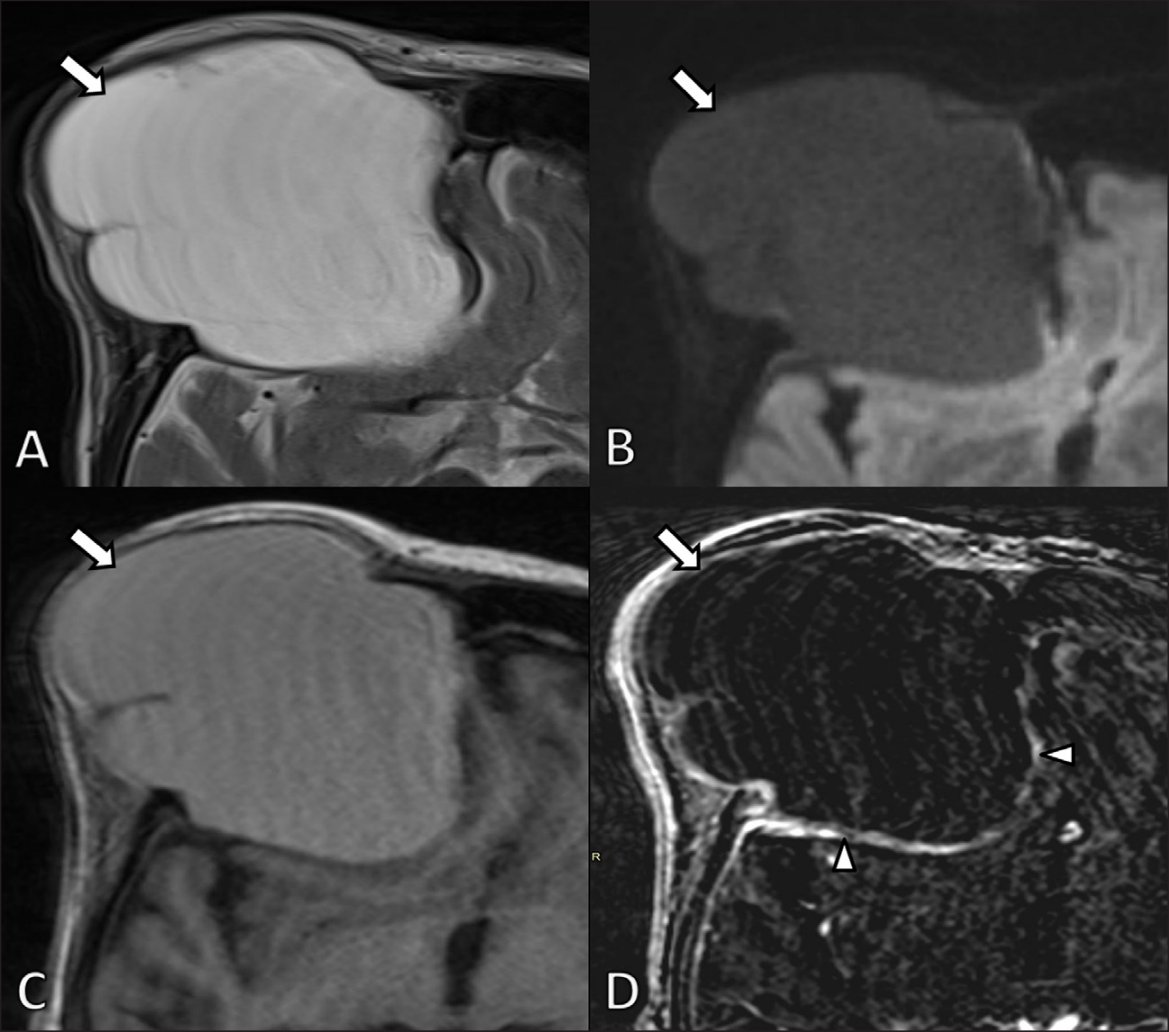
MRI scan 4 years later. A. Axial T2-WI. B. Diffusion weighted image (b1000) and C T1-WI and D. subtraction image of T1-WI before and after gadolinium contrast administration. The lesion (arrows) is slightly more hyperintense on T2-WI and slightly less intense on T1-WI compared to the previous examination due to a higher fluid content. There is no diffusion restriction and persistent subtle peripheral contrast enhancement (arrowheads).
